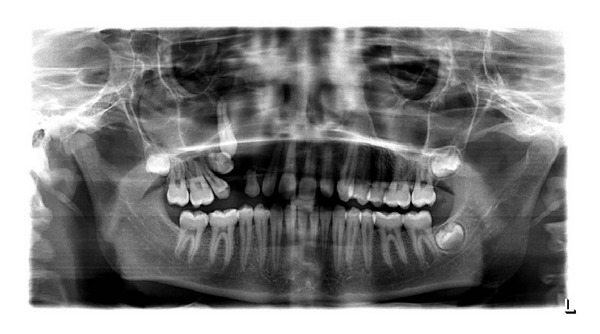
Panoramic radiograph showing impacted 13, 14.
